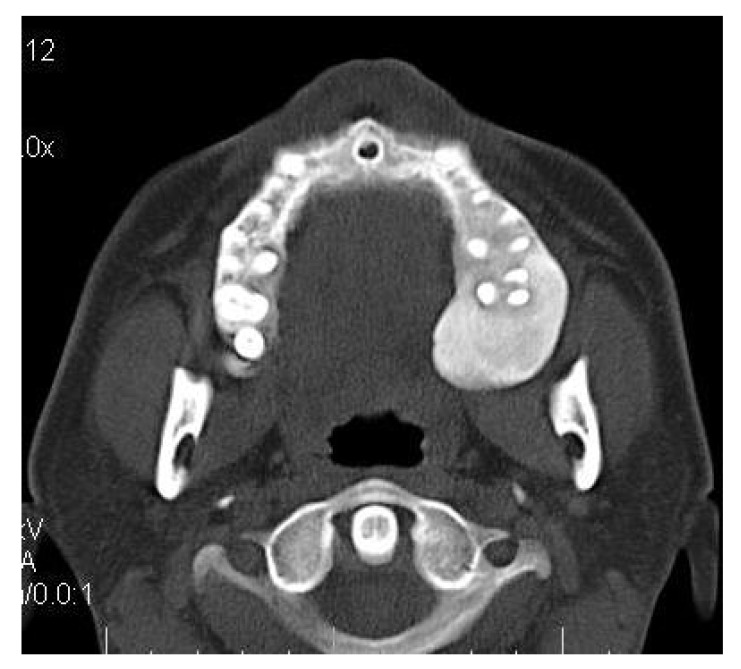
Axial CT section revealing involvement of left maxillary alveolus and tuberosity.
